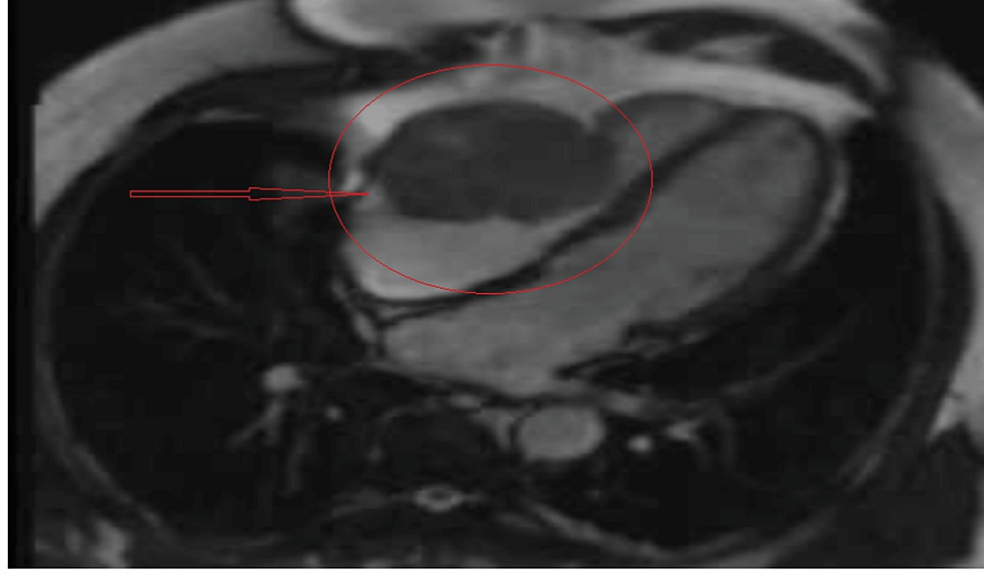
The cardiac MRI shows a right ventricular mass
